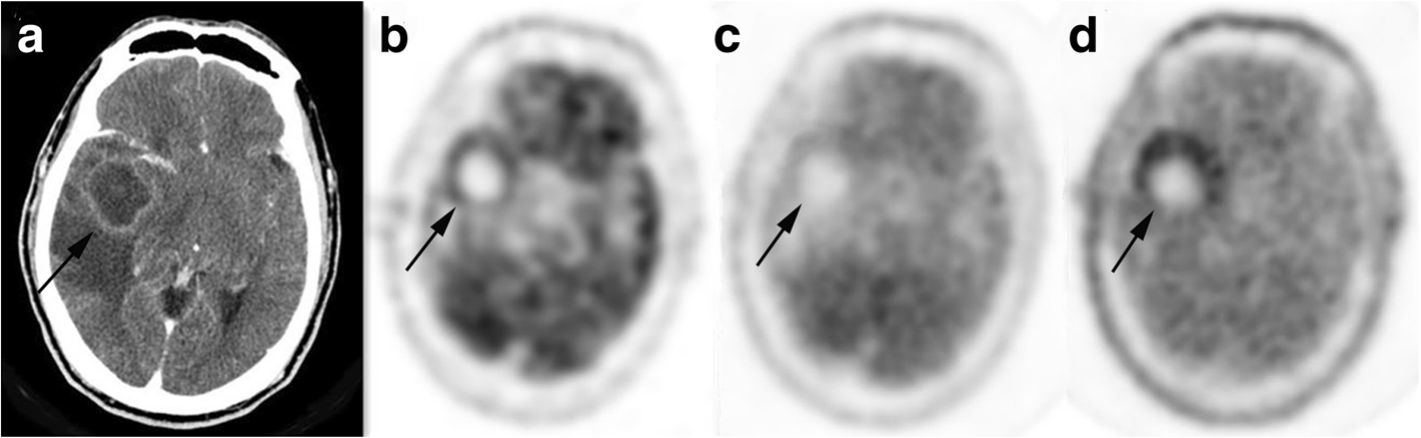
A patient aged 50–55 years with brain abscess. The lesion displays obviously increased uptake of ^11^C-MET (d) and mild increased uptake of ^18^F-FDG (b) with lower uptake of ^13^N-NH_3_ (c) compared with contralateral normal brain parenchyma. Contrast CT (a) shows a ring enhanced lesion in the right temporal lobe (arrow)
